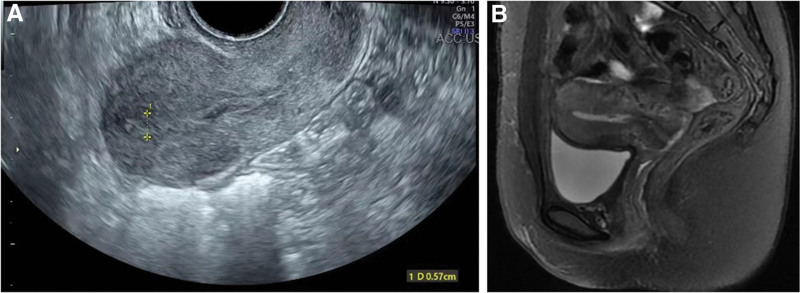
Pelvic TVS (A) and MRI (B) of the uterus show normal endometrium and myometrium 3 months after the operation (case 2). MRI = magnetic resonance imaging, TVS = transvaginal ultrasonography.
